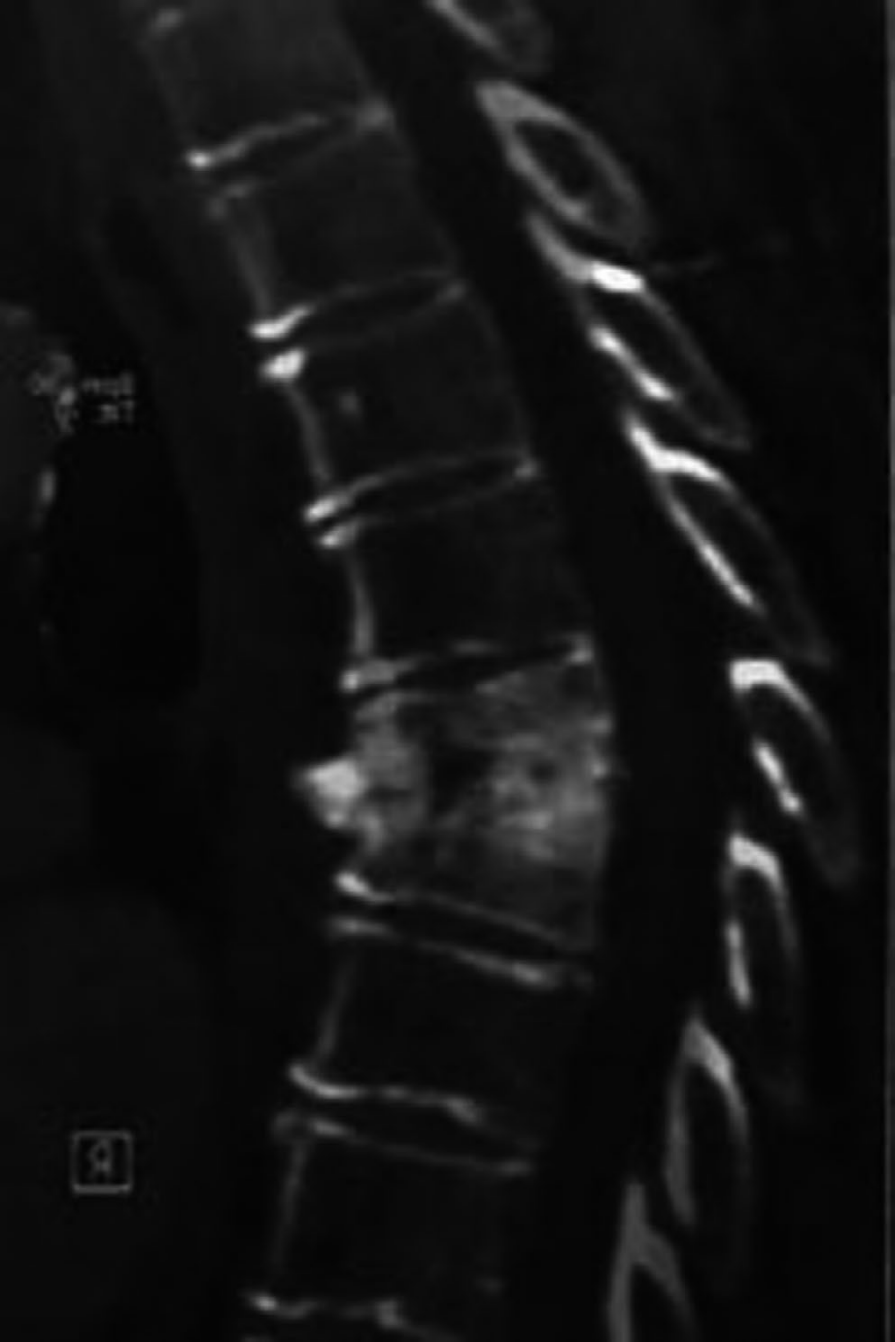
Thoracic sagittal T1‐weighted MRI image shows destruction and collapsed of the body of T6, T7 vertebrae
